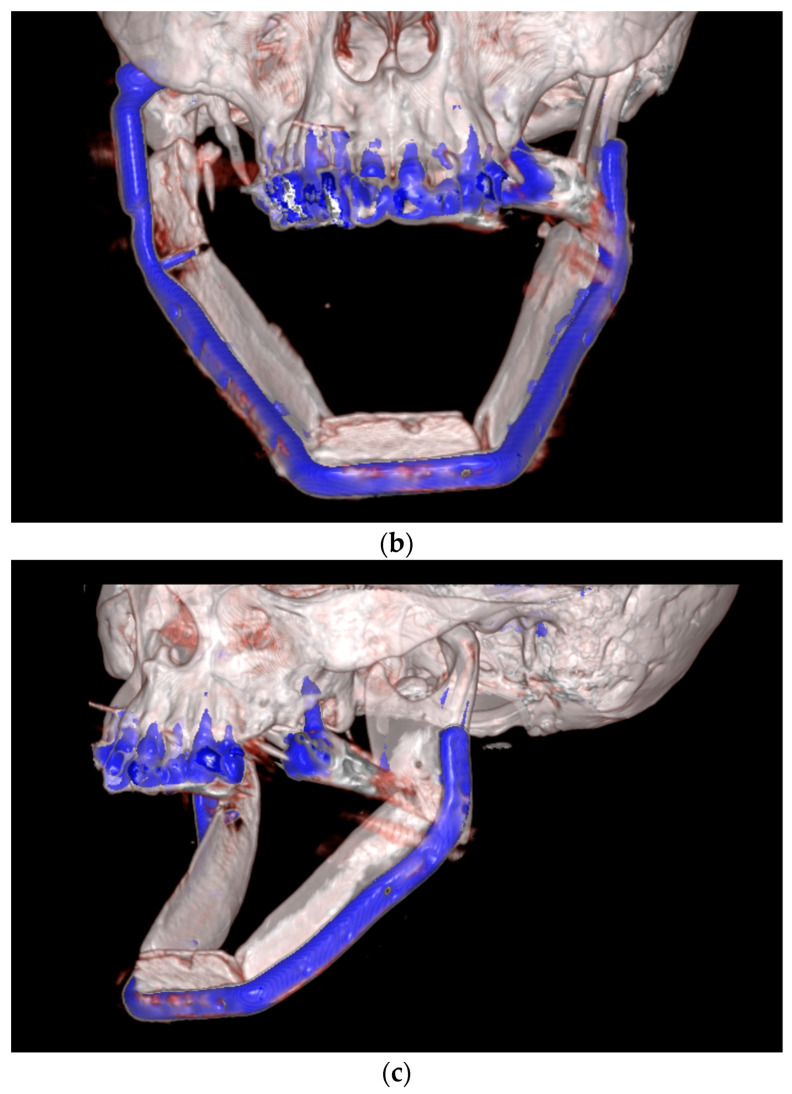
(a–c) shows the 3D reconstruction of the postoperative CT scan after resection and reconstruction of the mandible using free scapular flaps from both sides as virtually planned. The patient-specific implant is indicated in blue (along with the upper teeth).
